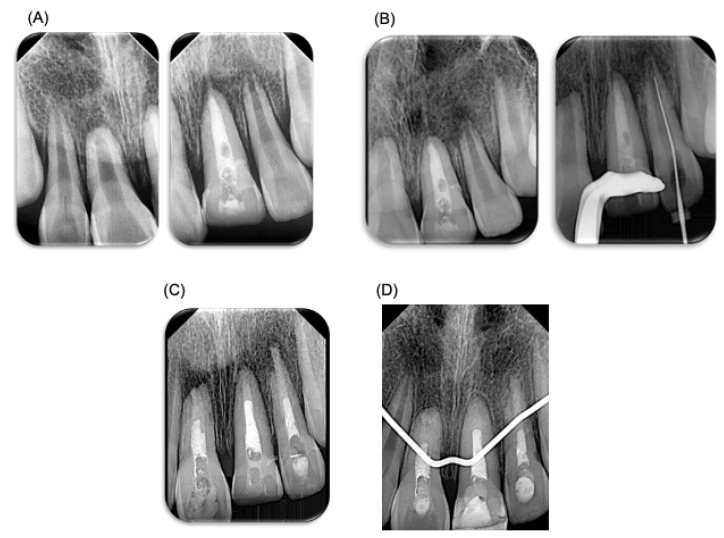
Endodontic treatment. Endodontic treatment performed on patient. (A) X-ray prior to endodontic treatment performed. (B) Control X-ray, 3 weeks after endodontic treatment, where root resorption can be observed. (C) X-ray after the performed endodontic treatment. (D) X-ray at 3 months, where the apical closure of teeth can be observed (teeth 11 and 21). A Biodentine^TM^ plug was placed. This material has a different radio-opacity than gutta-percha material; therefore, in the X-ray it may look like the endodontic treatment was not performed fully.
